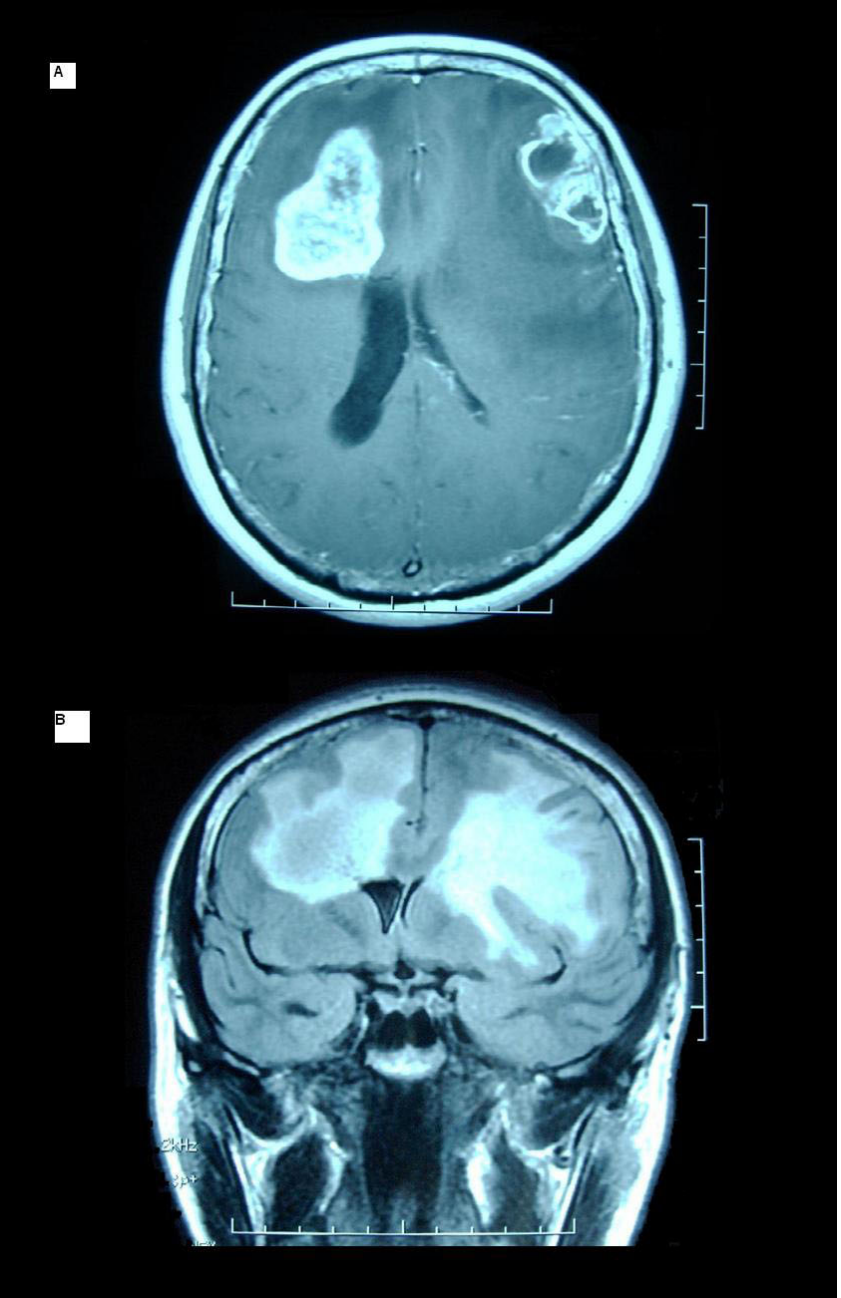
A – Preoperative T1gado-axial Magnetic Resonance Imaging; B – Preoperative FLAIR-coronal Magnetic Resonance Imaging.
